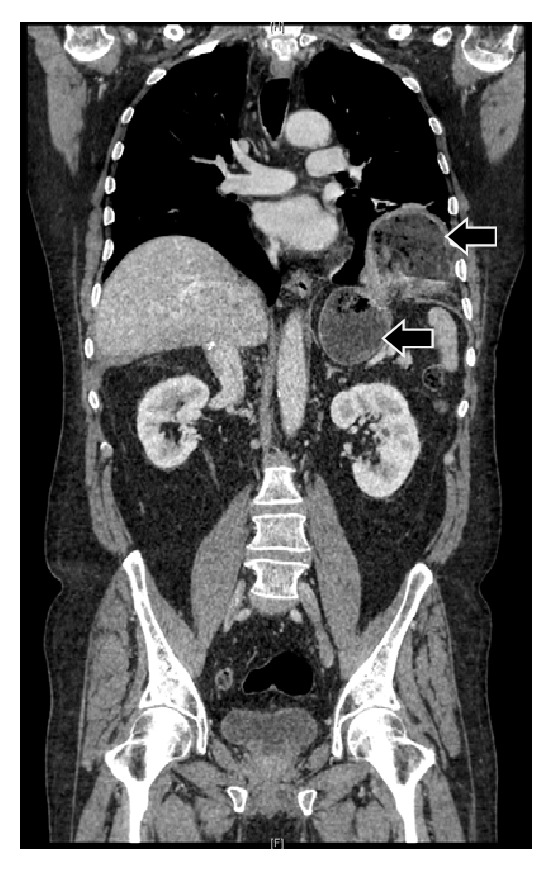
Abdominal CT scan (both arrows point at gastric content, the highest one into the thorax).
